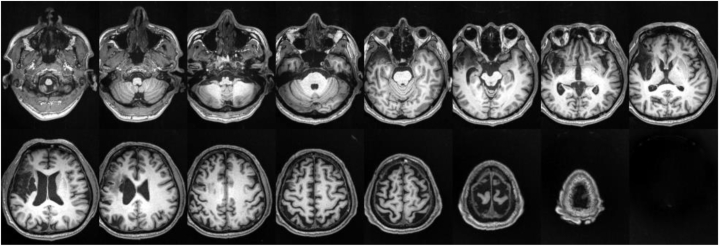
MRI of the patient, the brain lesion was predominantly located in his right hemisphere.
